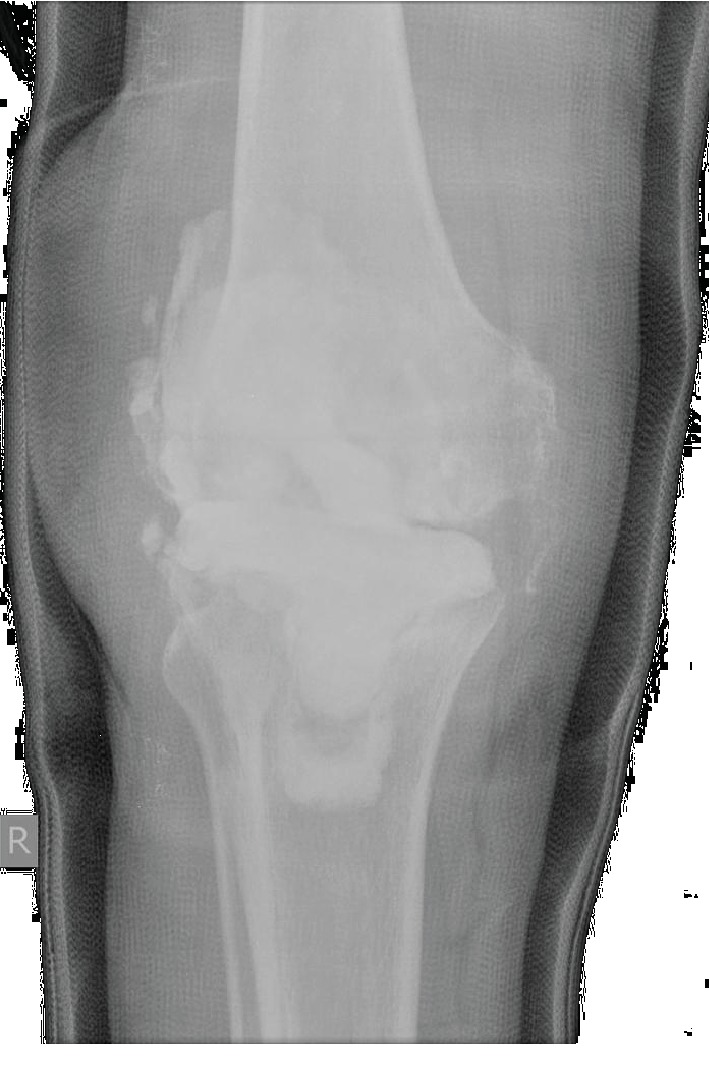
Radiograph shows cement spacer in situ.
